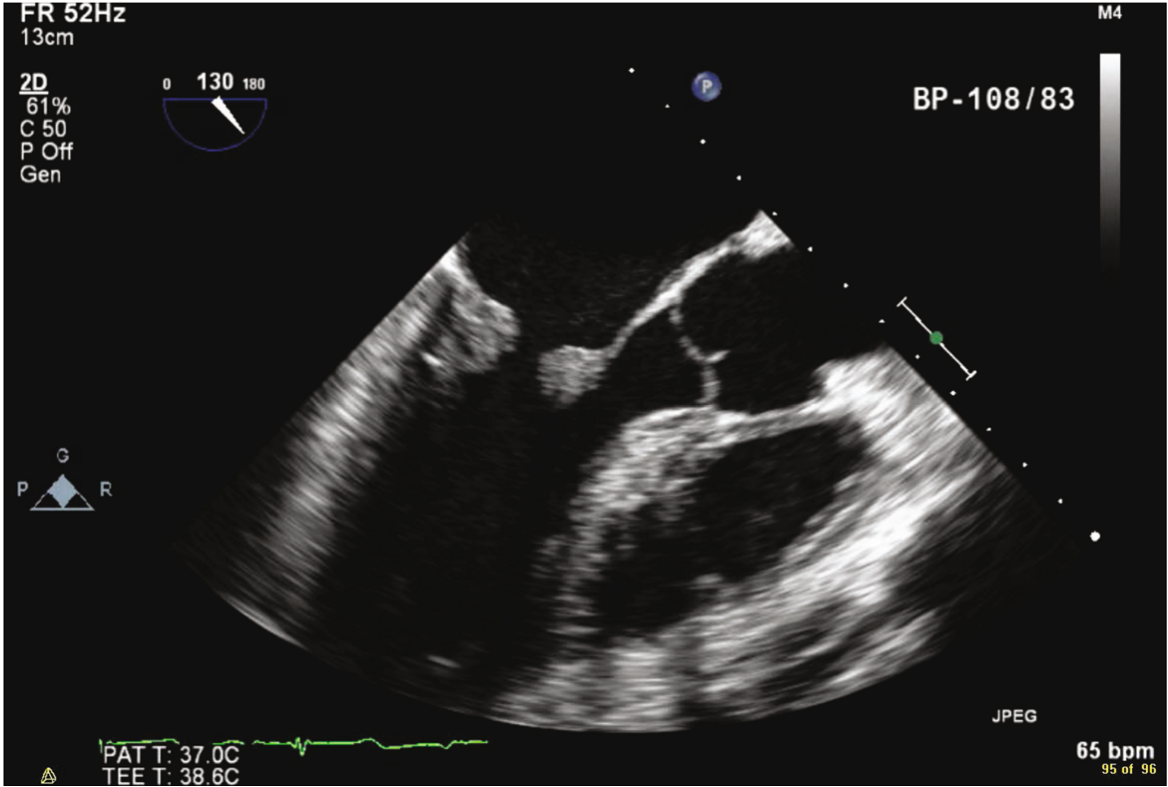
Transesophageal echocardiogram showing a symmetrical mass-like structure on the ventricular surface involving the tips of both leaflets extending to the body, causing a restriction in the valve function.
